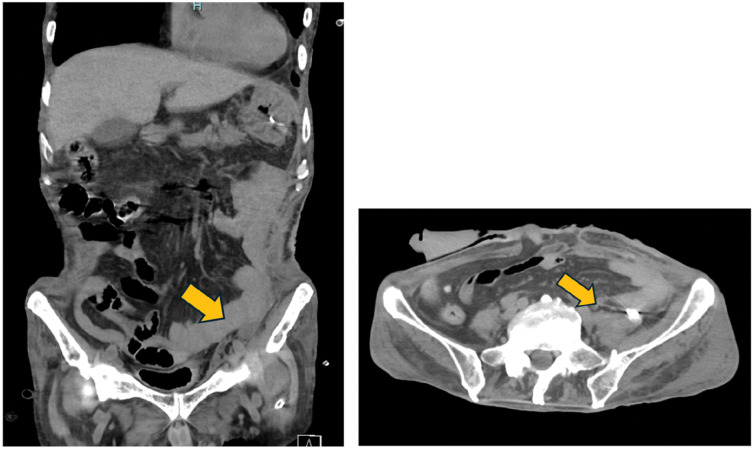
CT scan on POD 30. The yellow arrows show resolution of the abscesses in the abdominal cavity.
